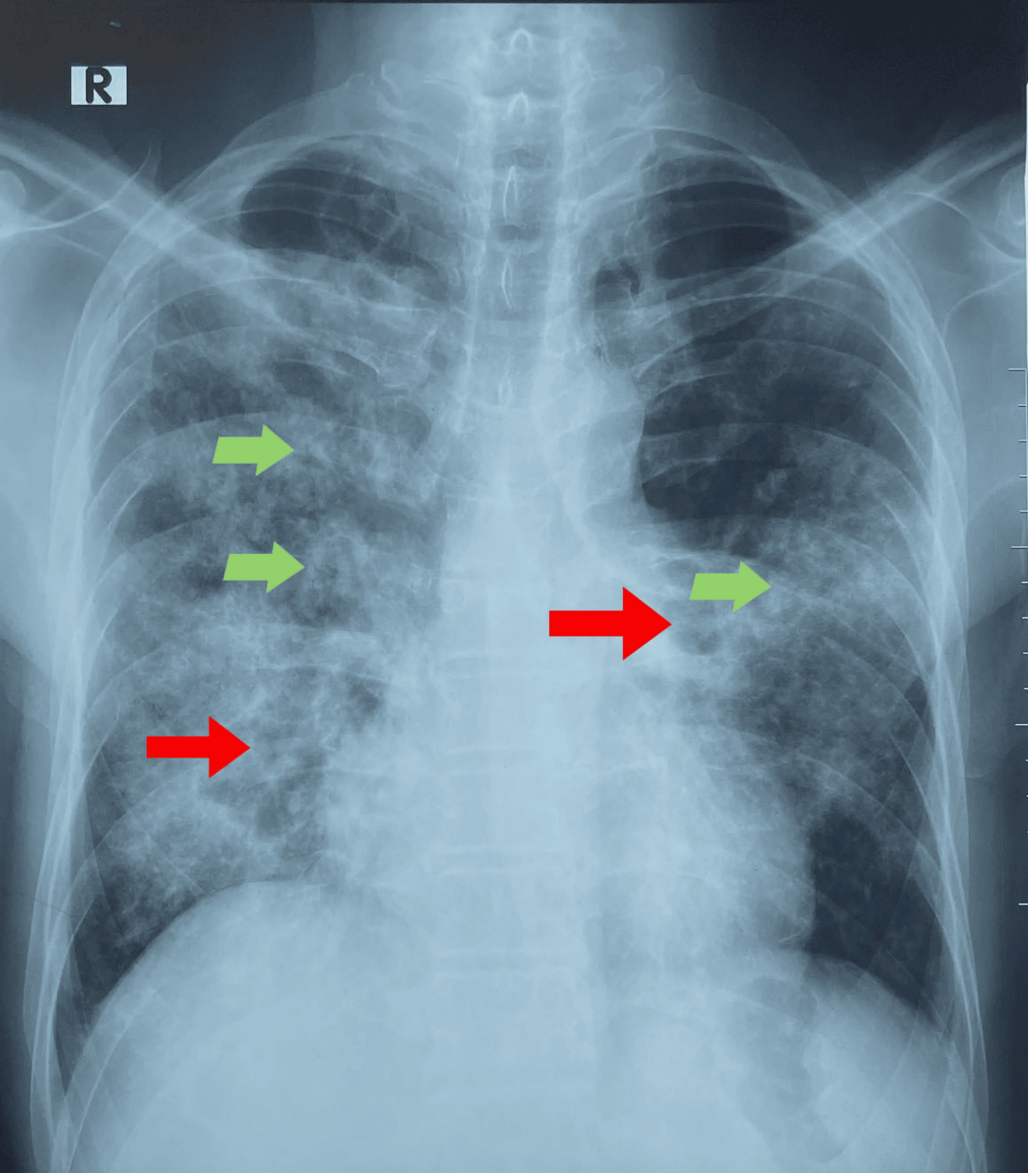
Chest X-ray of the patient X-ray of the chest revealed bilateral (B/L) multiple cysts (red arrows) and nodular lesions (light green arrows).
